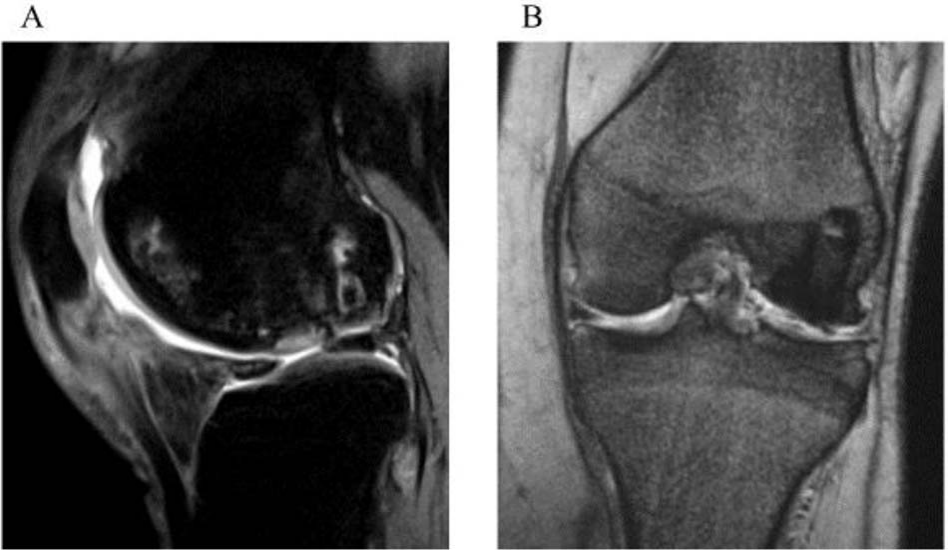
Two-year postoperative MRI. A sagittal fat saturation T2-weighted image (a) and a coronal T2-weighted image (b) show smooth articular surface in the grafted area and consolidation of the transplanted bone plugs.
